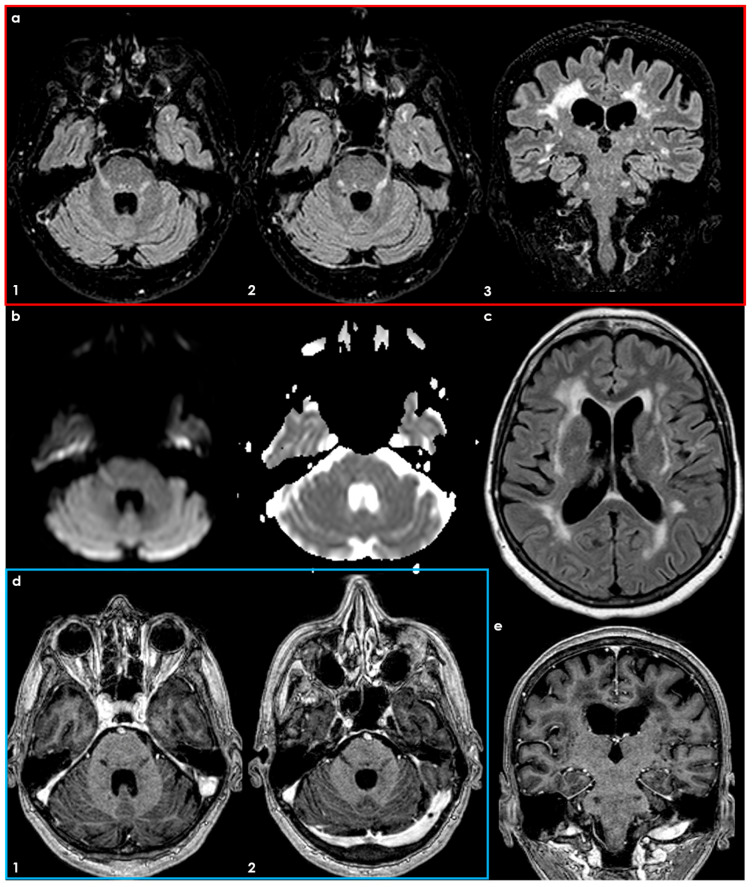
Patient with VZV recurrent infections: Axial panel (a1,a2) and coronal panel (a3) FLAIR MRI showing a bilateral trigeminal pontine sign. In panel (b), DWI and ADC slices at the same level. In panel (c), a supratentorial small vessel disease pattern is detailed with white-matter hyperintensities in the supratentorial location. In panels (d,e), the axial and coronal post-contrast T1W-MRI shows the lack of contrast enhancement of the pontine lesion.
